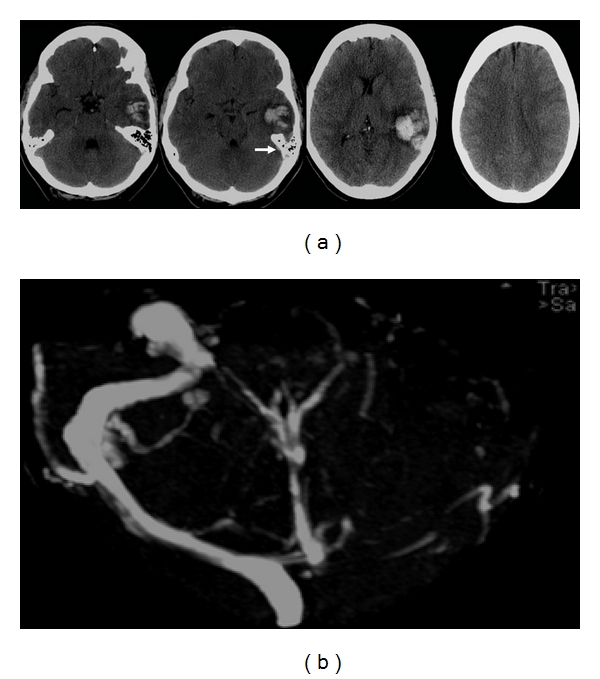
43-year-old woman presented with acute confusion with no history of trauma. (a) Axial unenhanced CT images show a large hemorrhage centered in the left temporal lobe. The subtle hyperdensity in the left sigmoid sinus is suggestive of thrombus (arrow). The topography of the lesion is not what would be expected from a hemorrhagic infarct involving the inferior division of the middle cerebral artery, that is, the surrounding hypodensity fails to reach the cortical surface. (b) MR venogram shows occlusion of the left transverse and sigmoid sinuses, confirming the hemorrhage is secondary to venous infarction.
